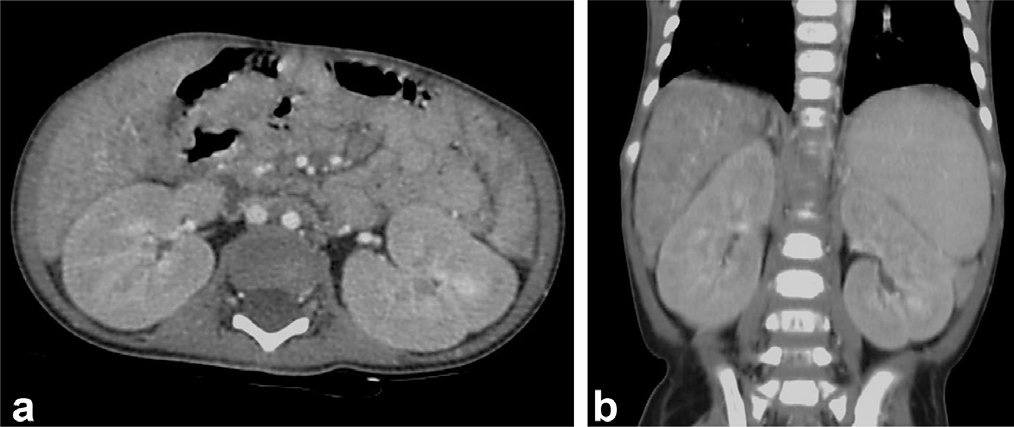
(a) CT scan, post-contrast, axial images demonstrate hypodense kidneys with indistinct corticomedullary distinction. (b) CT scan, coronal images shows large-sized hypodense kidneys.
